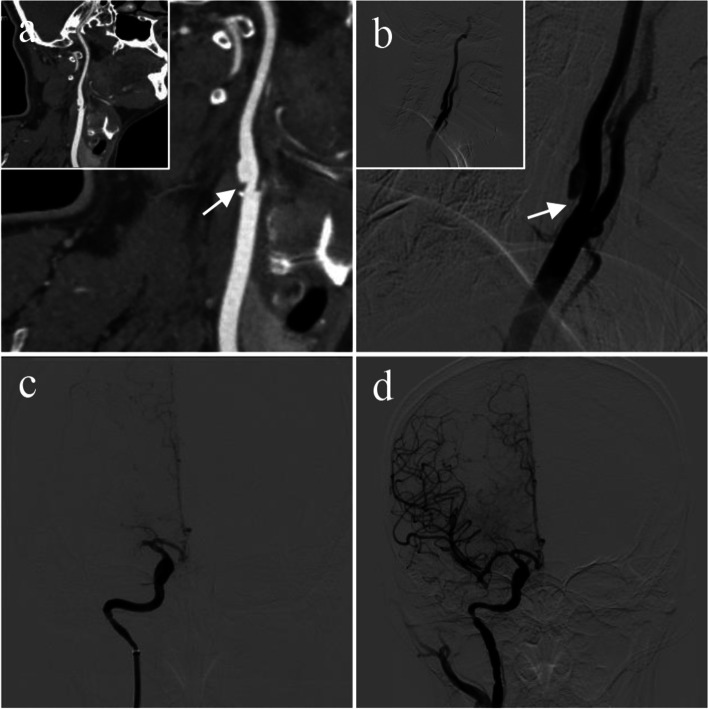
a, b CTA and DSA images of carotid artery webs showing a thin, membrane-like structure (white arrow) in the carotid bulb. c A right internal carotid angiogram demonstrating MCA occlusion. d Mechanical thrombectomy achieved recanalization of the right MCA
